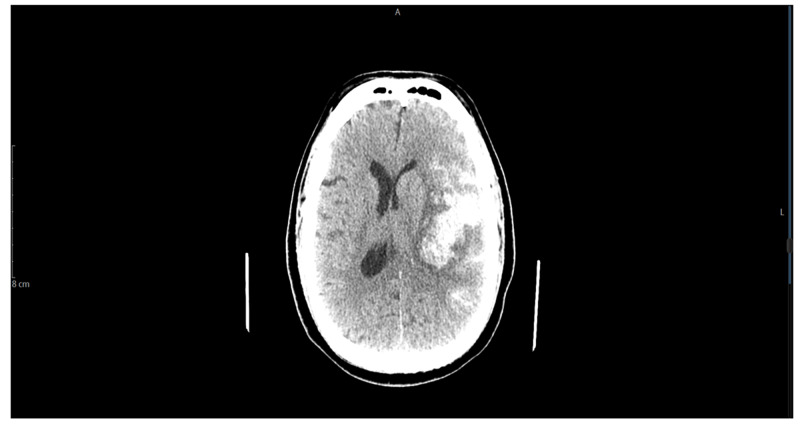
Non-contrast CT of the head showed a large intraparenchymal hemorrhage in the left external capsule, peri-insular region with subarachnoid hemorrhage CT: computed tomography
